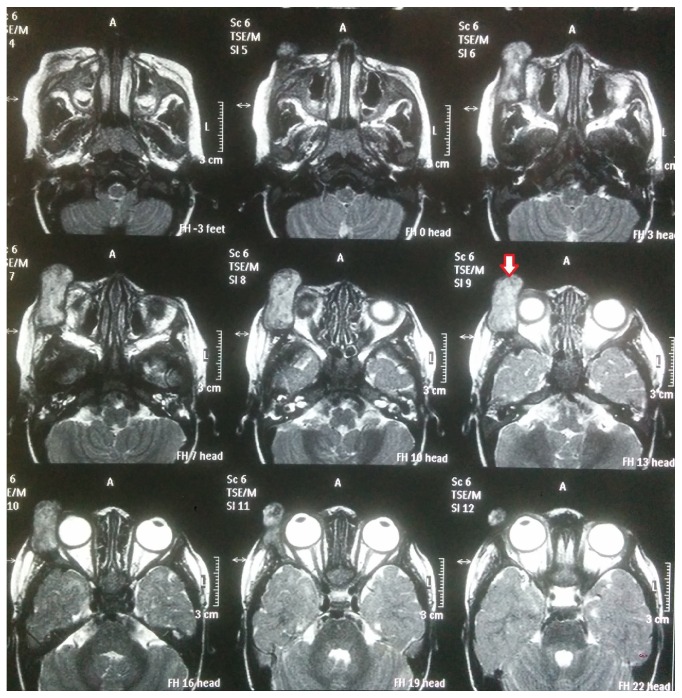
Prechemotherapy MRI scan showing localization and extent of eosinophilic granuloma with bony erosion. Arrow shows the actual pathology, that is, the eosinophilic granuloma.
